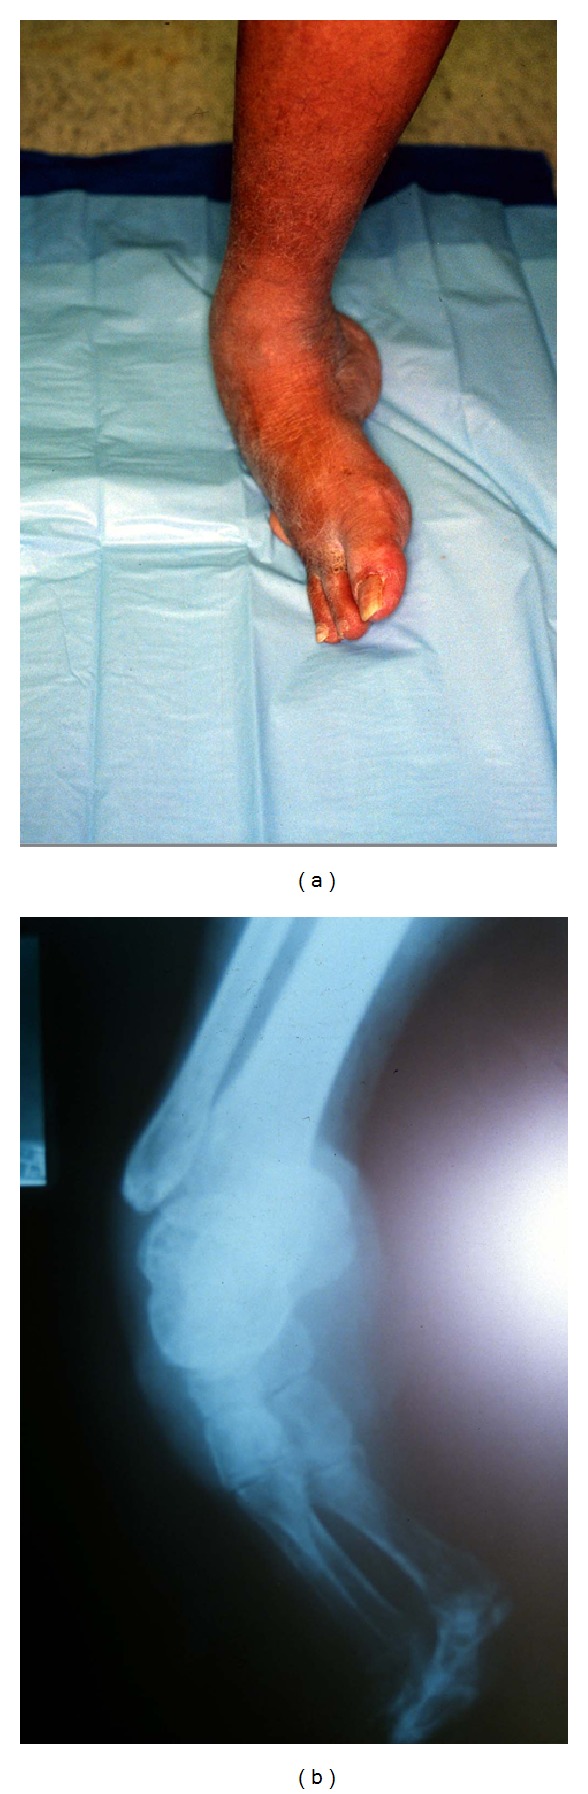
(a) Overpowering of the extrinsic foot muscles leading to an equinus deformity at the ankle and a varus hindfoot. (b) X-ray image showing ankle and foot deformity.
